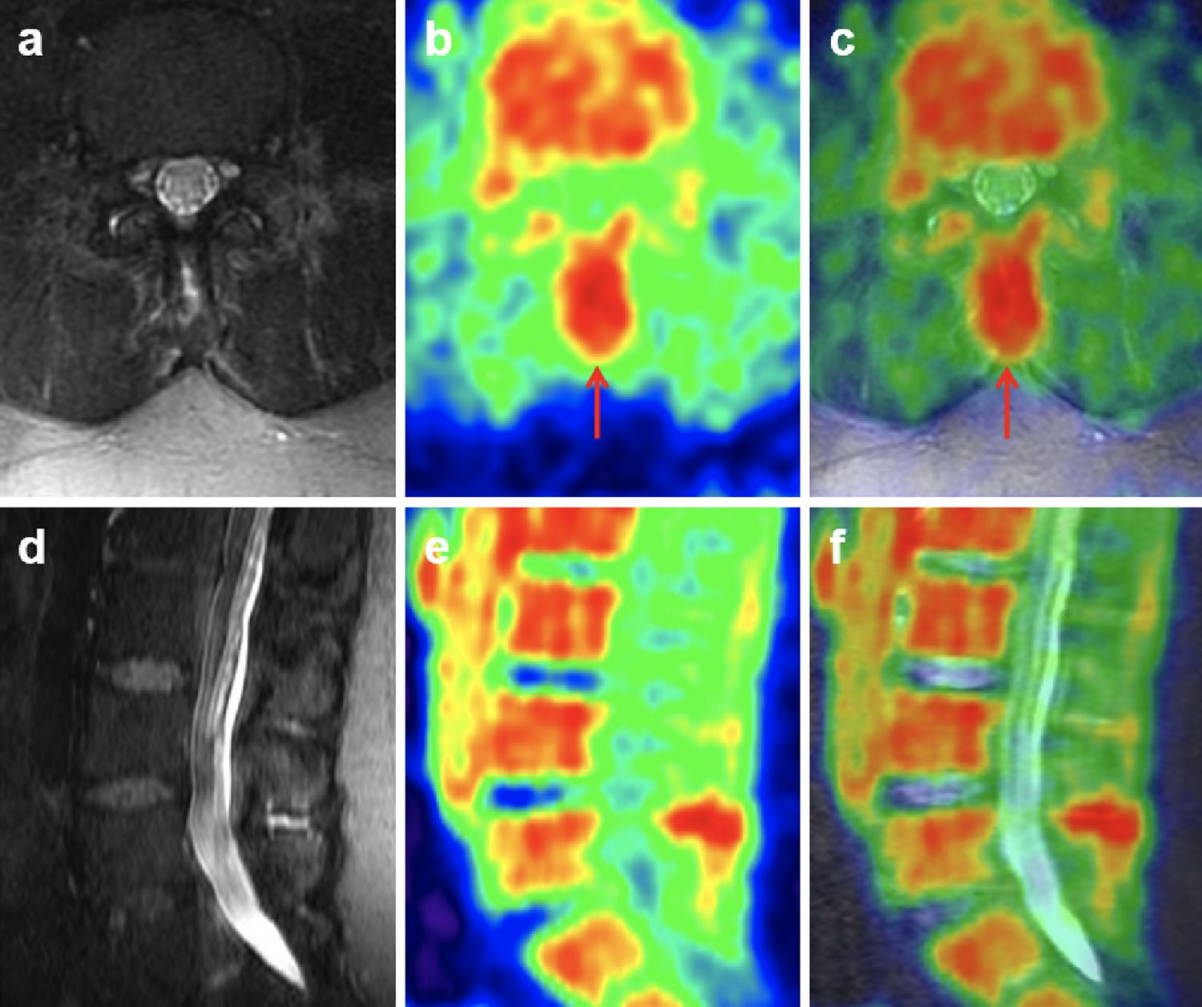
[18F]FDG PET/MRI abnormalities at L4-5 at level of interspinous ligament of patient with suspected cerebrospinal fluid leak, not observed on conventional MRI. High resolution axial MSDE-UBE MRI (a) shows increased signal in the vicinity of the interspinous ligament and paraspinal muscles, which was not observed in the original conventional lumbar spine MRI the patient had recently before this PET/MRI. Axial [18F]FDG PET (b) and PET/MRI (c) shows increased radiotracer uptake posterior to the spinal canal in the region of the interspinous ligament (red arrow, SUV_max_ 3.25). Sagittal reformats of the MSDE-CUBE MRI, PET, and PET/MRI are shown in (d), (e), and (f), respectively to help appreciating the abnormally increased uptake in this level compared to those in other levels.
